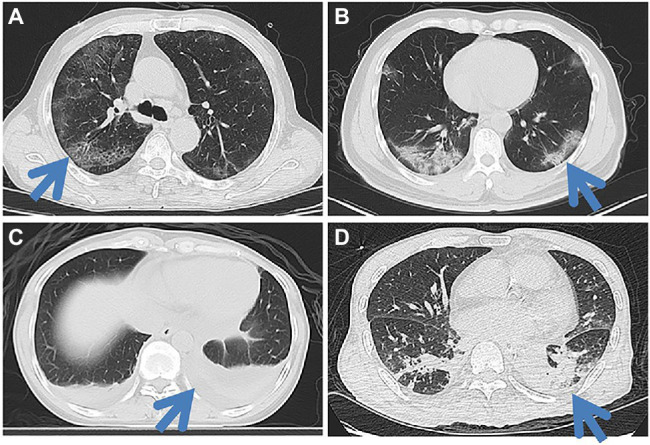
Typical CT imaging features in both COVID-19 patients (A,B) and influenza pneumonia patients (C,D). A 65-year-old man with COVID-19 (A) shows the crazy-paving pattern sign in the posterior segment of the right upper lobe along with bilateral peripheral multifocal ground-glass opacities (GGOs). A 46-year-old man with a COVID-19 (B) shows multifocal mixed GGOs in the lower lobe of both lungs, mainly in the peripheral. A 44-year-old female with influenza pneumonia shows lower lobe atelectasis in the posterior basal segment of both lungs, along with bilateral pleural effusions. A 60-year-old man with influenza pneumonia shows local consolidations in the posterior segment and lateral basal segment of both lower lobes.
